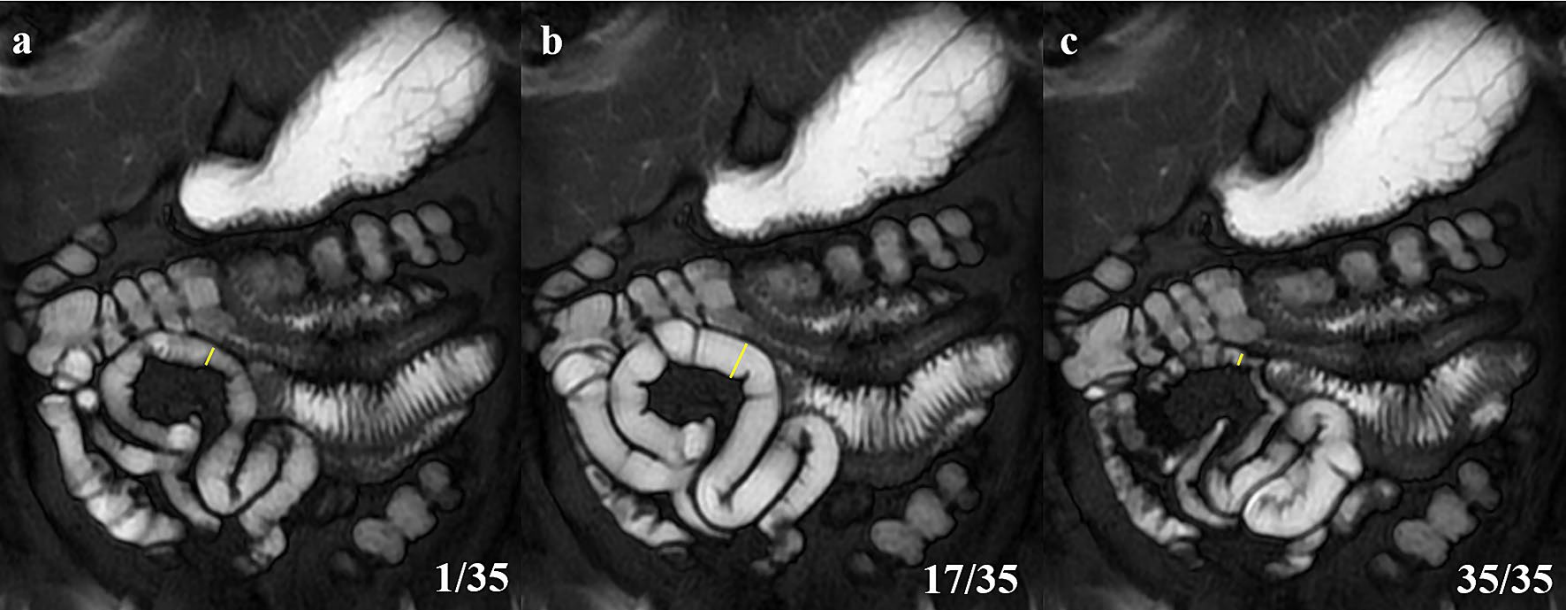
Luminal diameters of the small intestine (yellow line) are calculated using the software. This task was repeated for all sequential cine magnetic resonance imaging. The typical findings are shown as (a) (1st of the series), (b) (17th of the series), and (c) (35th of the series).
